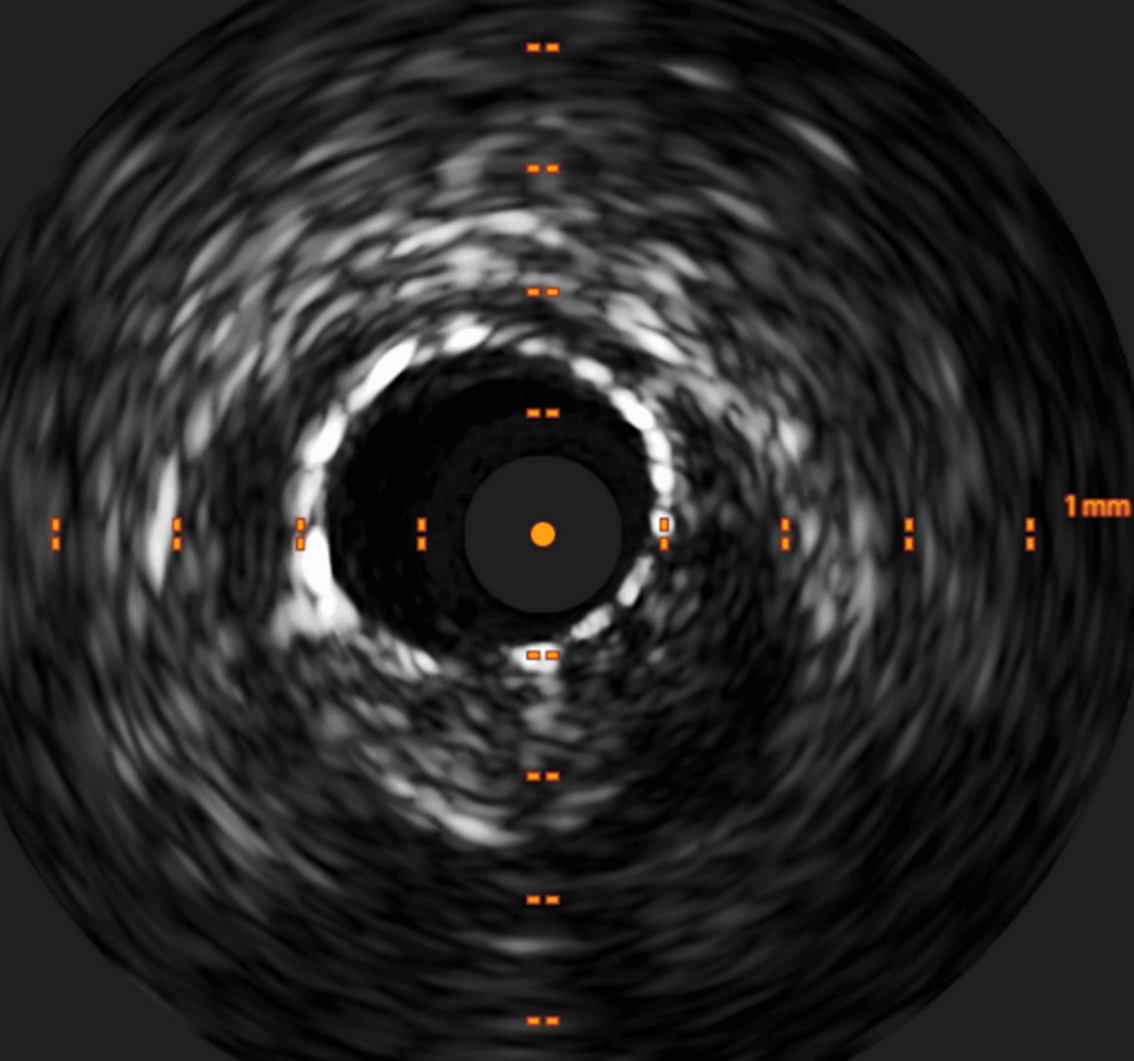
Intravascular ultrasound Optimal stent expansion and adequate stent strut apposition are demonstrated on ultrasound.
